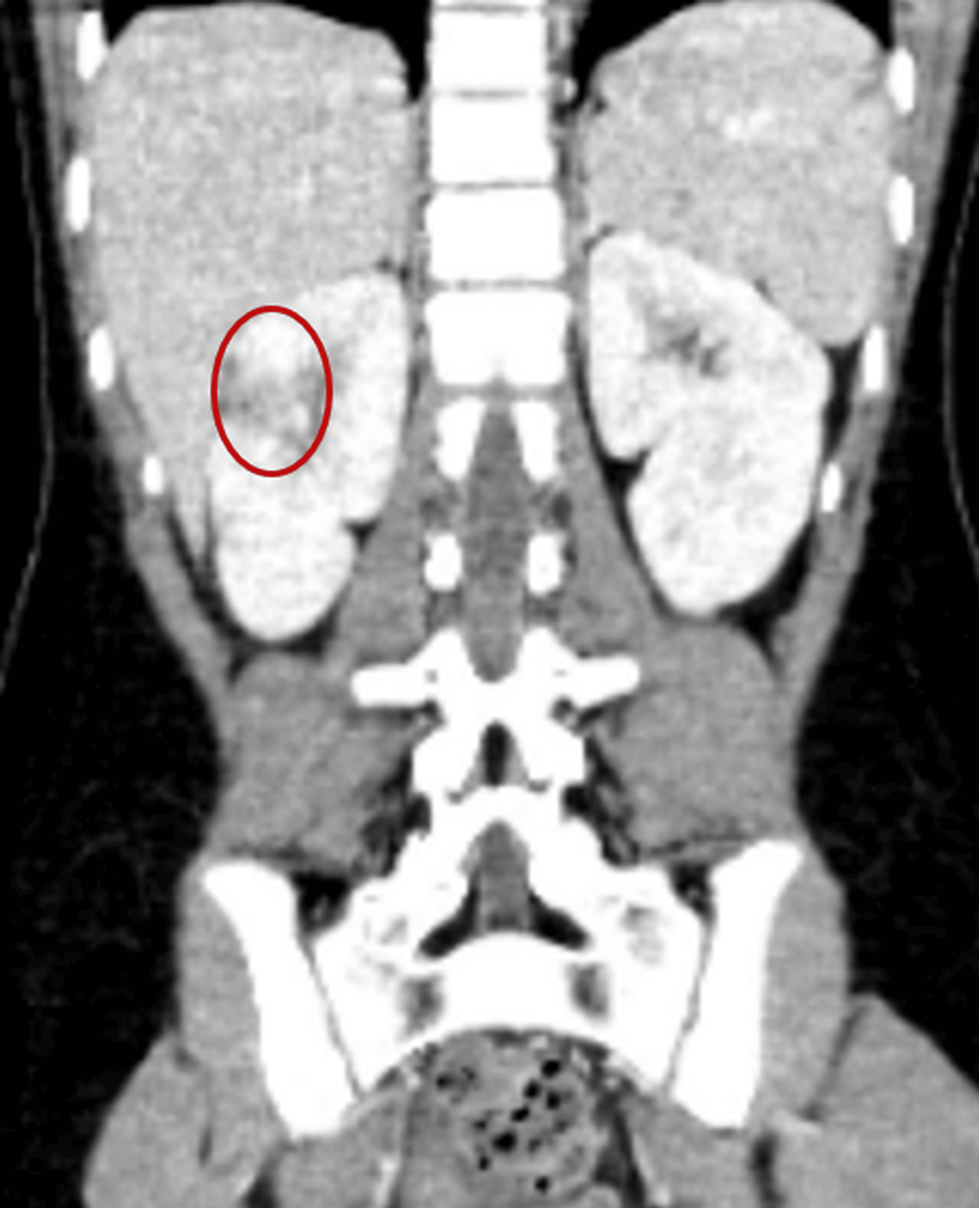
Coronal contrast-enhanced abdominal CT: right renal infarction (red circle) with a wedge-shaped hypodensity in the renal parenchyma
